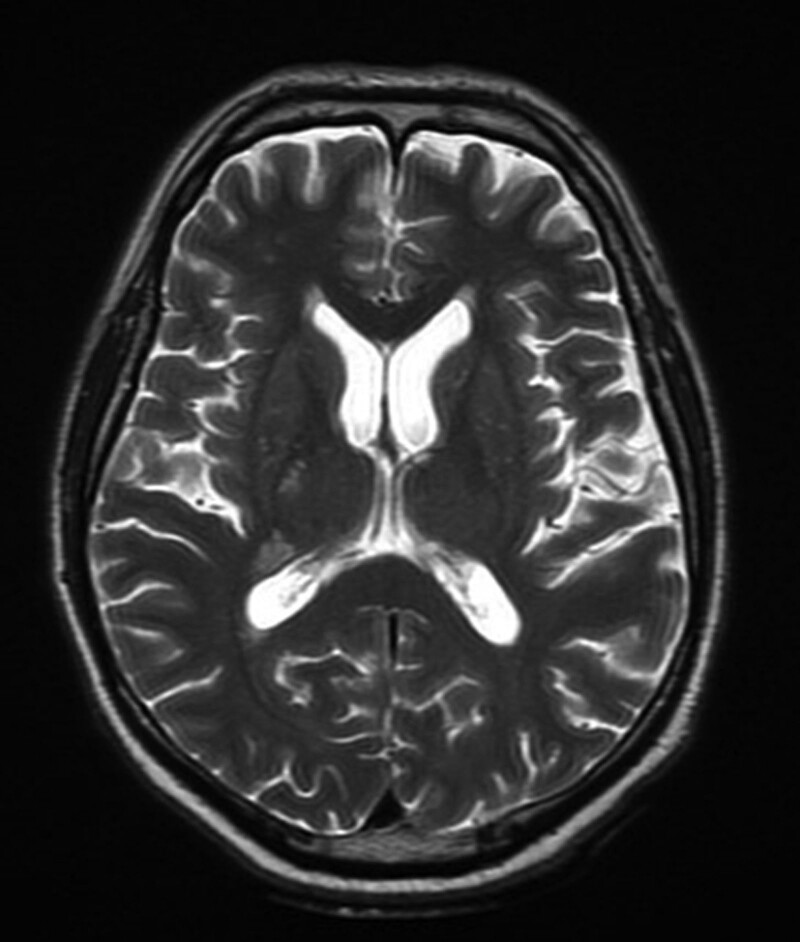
MRI scan of the brain with hyperintense foci of subacute ischemia in the deep structures of the right hemisphere (FLAIR sequence).
